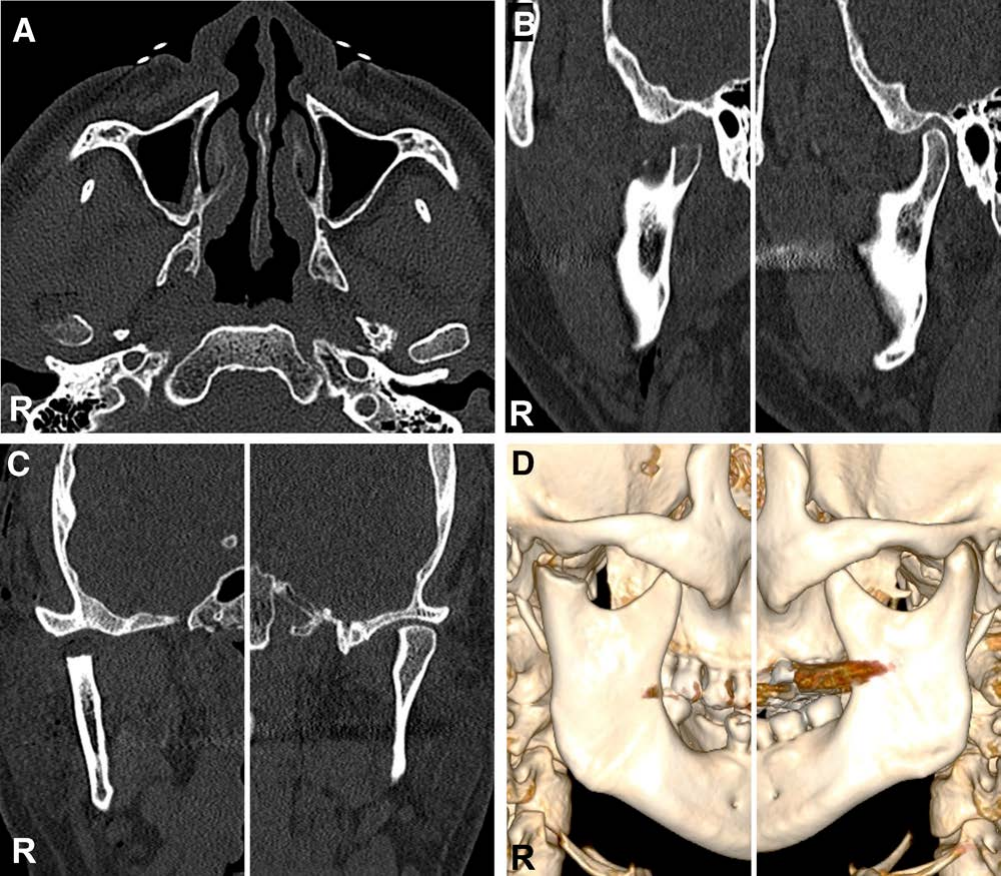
Immediate postoperative CT images. The lower part of the condylar neck was preserved. (A) Axial view. (B) Oblique sagittal view. (C) Coronal view. (D) 3-dimensional reconstruction. CT = computed tomography.
